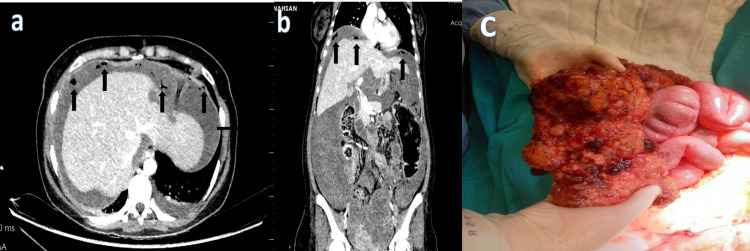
A 31-year-old female patient treated for metastatic colon cancer developed abdominal pain and distension, and free air (black arrows) was observed in the perihepatic area on dual-energy abdominopelvic CT with IV contrast. Perforation focus was not detected on CT examination. (a) Axial image. (b) Coronal image. (c) No perforation was found in the patient who underwent laparotomy due to free air in the abdomen.
